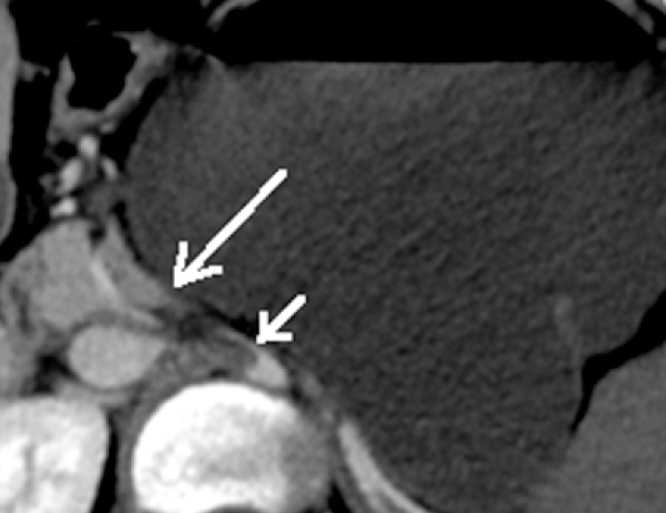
Patient 1. Preoperative CT. Compression of the pancreas (large arrow) and the coeliac trunk (arrow) from a massively dilated stomach.
